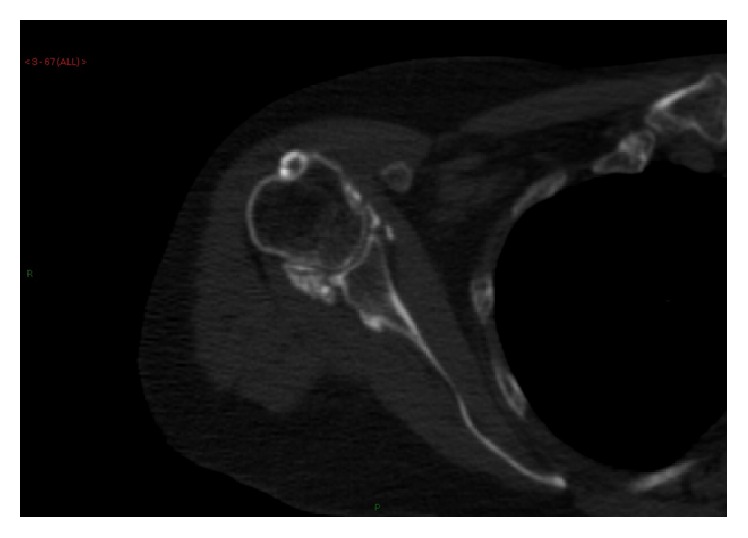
CT scan of the right shoulder confirmed intra- and extra-articular calcinosis.
